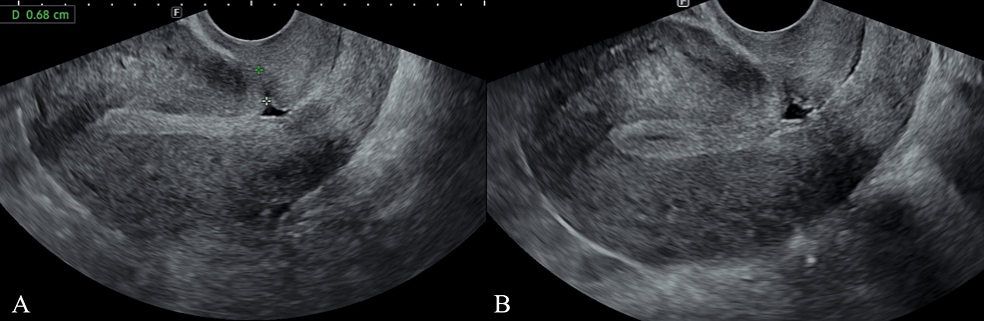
Transvaginal ultrasound images Transvaginal ultrasonography shows a cesarean scar defect with an RMT of 6.8 mm (A), as well as fluid retention in the uterine cavity (B). RMT: residual myometrial thickness
